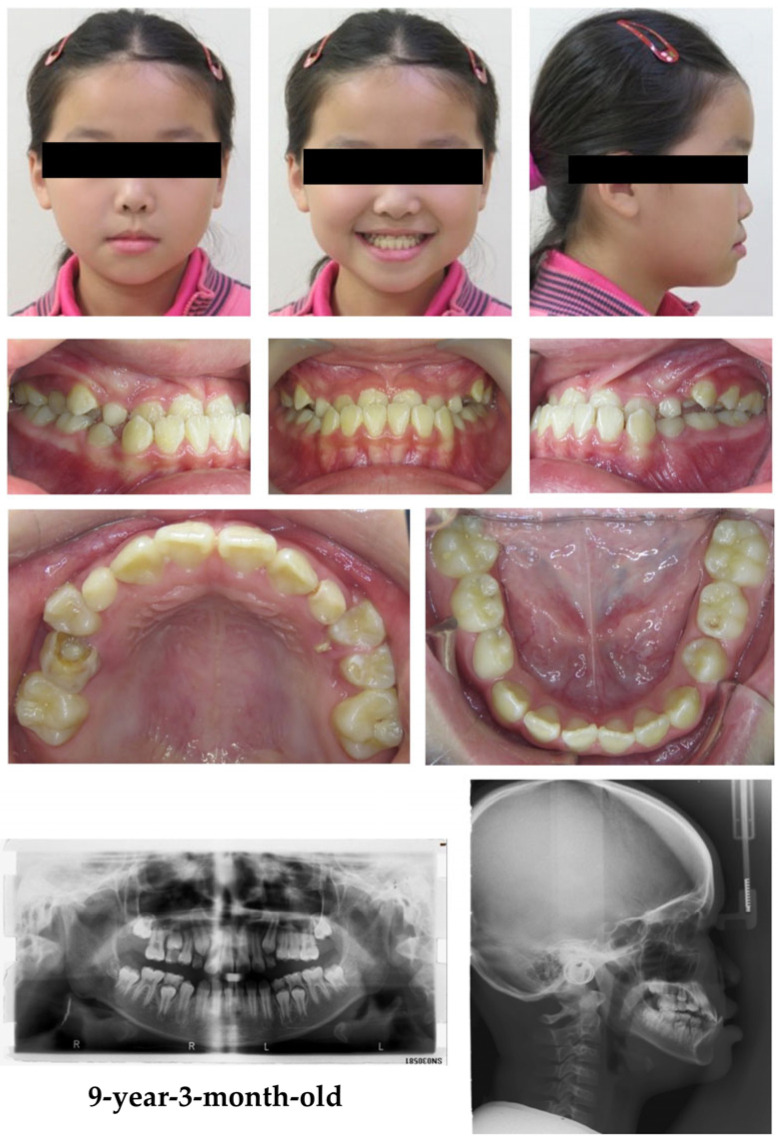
Pretreatment facial and intraoral photographs, panoramic radiograph, and cephalometric radiograph of a 9-year-3-month-old patient. The facial and intraoral photographs showed a protruding lower lip, reverse overjet, and a concave profile. The panoramic radiograph demonstrated mixed dentition, and the cephalometric radiograph indicated skeletal class III malocclusion and cervical vertebral maturation stage 2.
